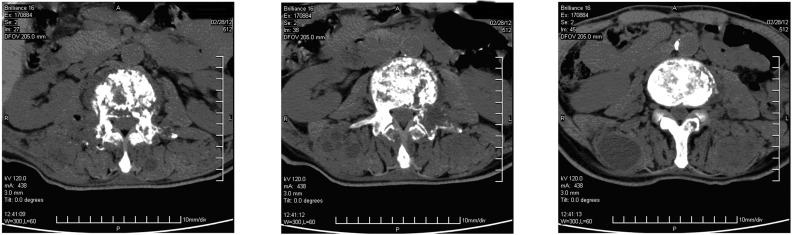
Computed tomography (CT) scan showing multiple myelomata. Chest 12/waist 1 vertebral level spinal stenosis.
